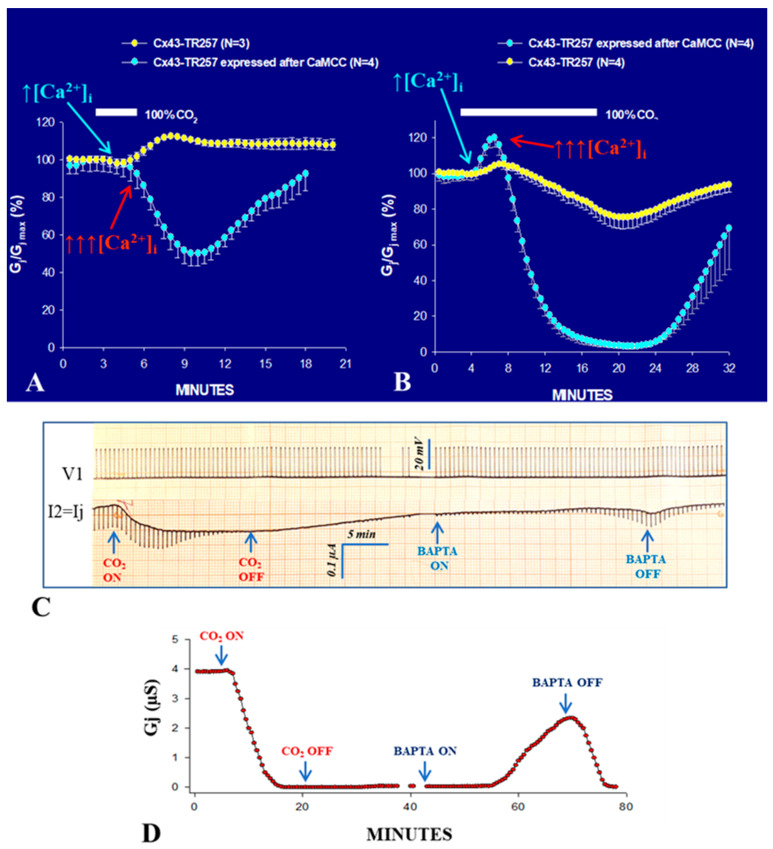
CT-deleted Cx43 channels (Cx43TR257), expressed after CaMCC, are much more sensitive to CO_2_ than controls (A and B), as Gj rapidly drops to ~50% (A) and nearly 0% (B) with 3 and 15 min 100% CO_2_ applications, respectively, while in controls Gj decreases by only ~25% even with 15 min CO_2_ (B). Curiously, in controls paradoxically Gj increases reversibly with brief applications of CO_2_ (A); similarly, Gj increases before dropping in control Cx43TR257 (B). The initial Gj rise may result from activation of the CaMKII cascade (see text). After CO_2_-washout, in some experiments, Gj remains indefinitely at nearly 0% (C,D), but reversibly increases with 180 µM BAPTA superfusion (C,D). An original voltage (V1 = Vj) and current (I2 = Ij) chart record is shown in C. The changes in Gj (µS) of the experiment shown in C are shown in D (Gj = Ij/Vj). These Cx43 data are mine, new and not published elsewhere.
